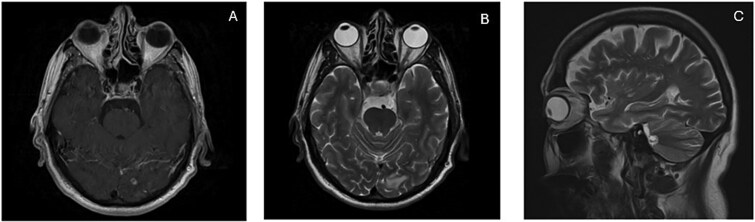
(A & B) MRI brain with and without contrast demonstrating occipital lobe deposit with surrounding edema. (C) Progressive disease with new deposit at cerebellar vermis.
